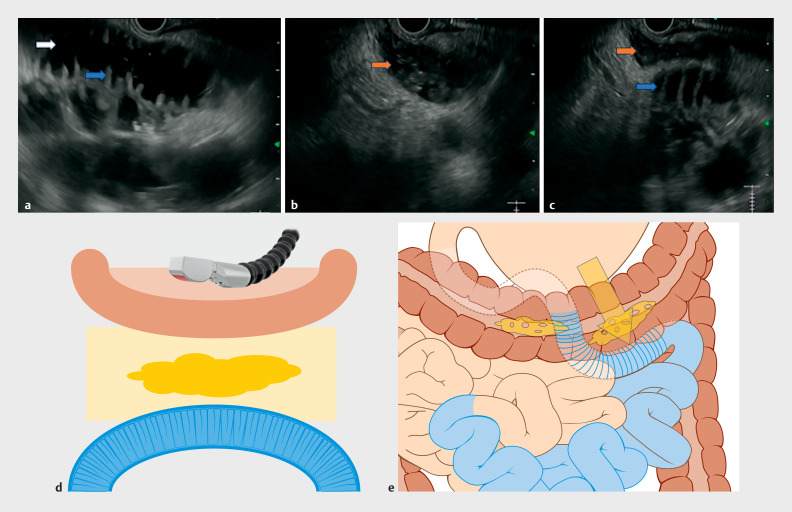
Features of the intestinal structures on endoscopic ultrasound.
